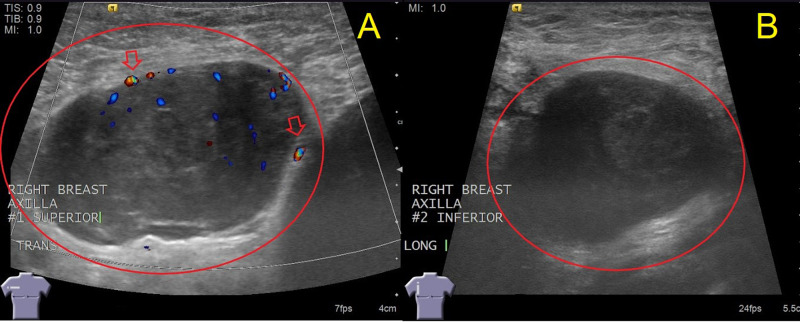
Right Axilla Ultrasound Images show a 37 mm x 24 mm x 40 mm irregularly shaped axillary lymph node (A) with an associated increased vascularity (arrows) and a 35 mm x 31 mm x 39 mm irregularly shaped adjacent lymph node (B) immediately inferior to lymph node A. Irregular lymph nodes are indicated with red circles.
